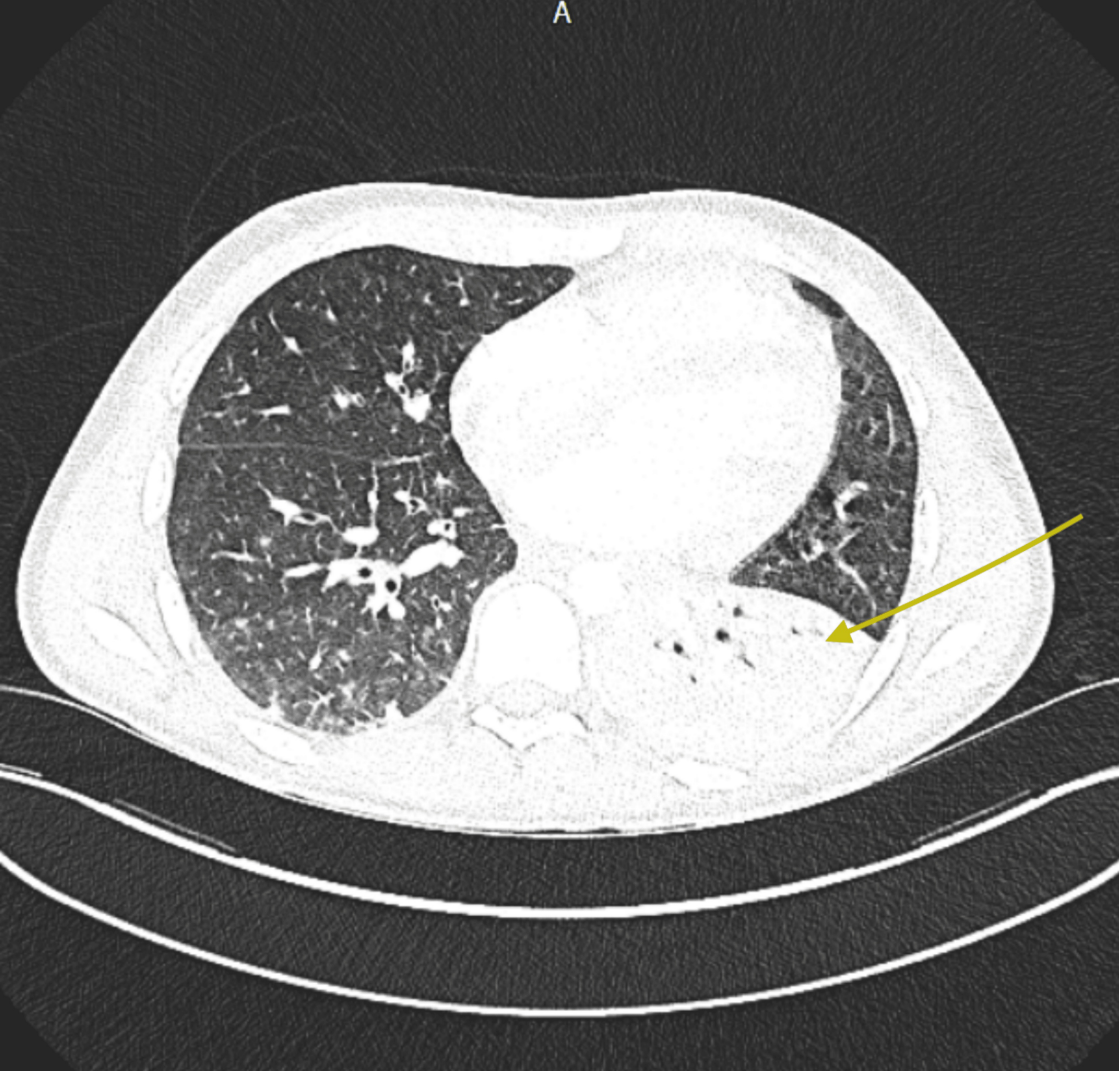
CTPA demonstrating left lobar pneumonia CTPA: computed tomography pulmonary angiogram
